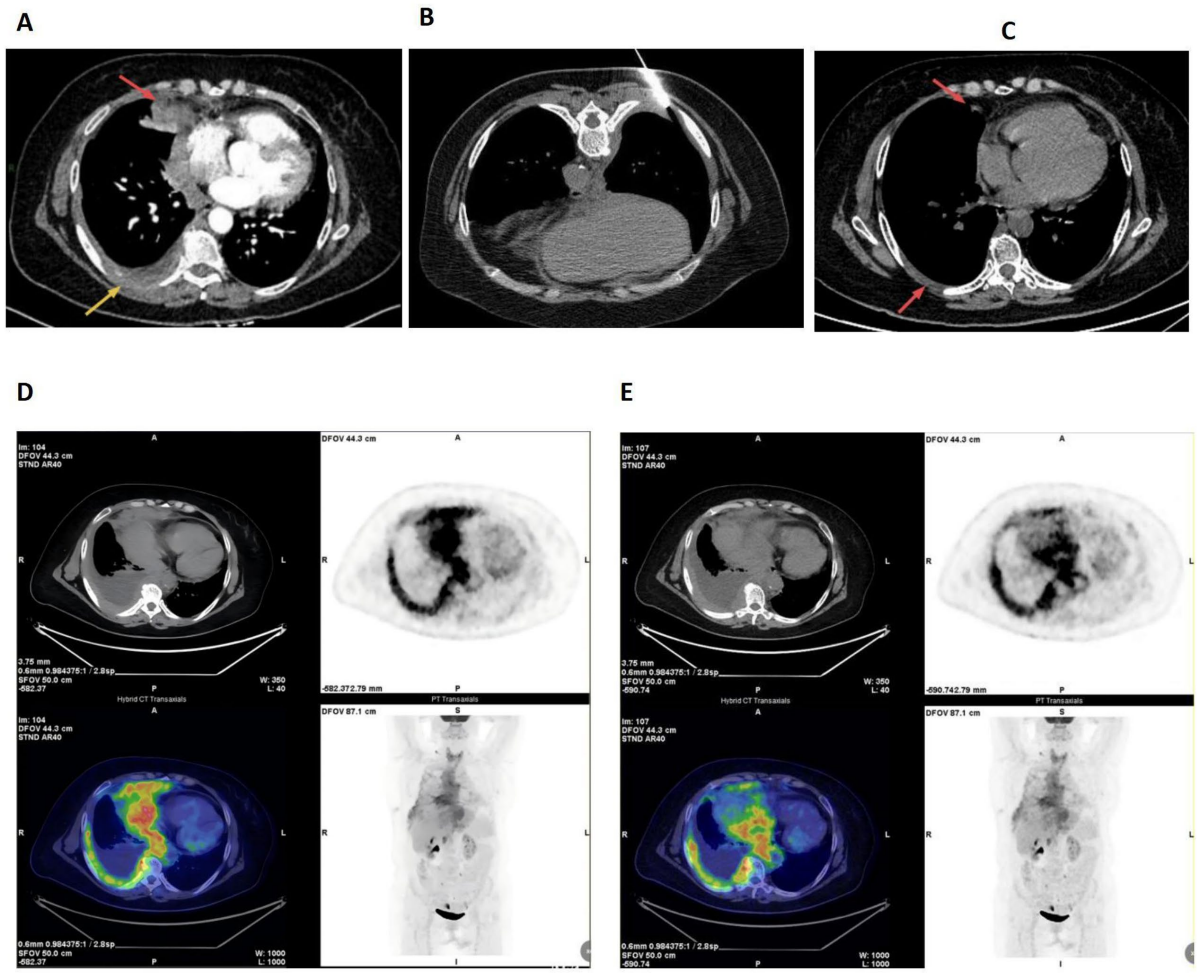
Relevant examination results. (A) Enhanced chest CT images on December 23, 2024: Irregular mass located nearby right lung(red arrow), and the right pleura shows thickening(yellow arrow). (B) CT-guided percutaneous pleura biopsy on December 24, 2024. (C) CT images after chemotherapy on February 3, 2025: Nodules and thickening of the right pleura were significantly reduced (red arrow). (D,E) Whole body fluorodeoxyglucose (FDG) positron emission tomography-computed tomography (PET-CT) (PET/CT) images on January 6, 2025: B-LBL involved the right pleura and mediastinum regions. D Chest and ediastinum involvement, E Sub-diaphragmatic involvement.
